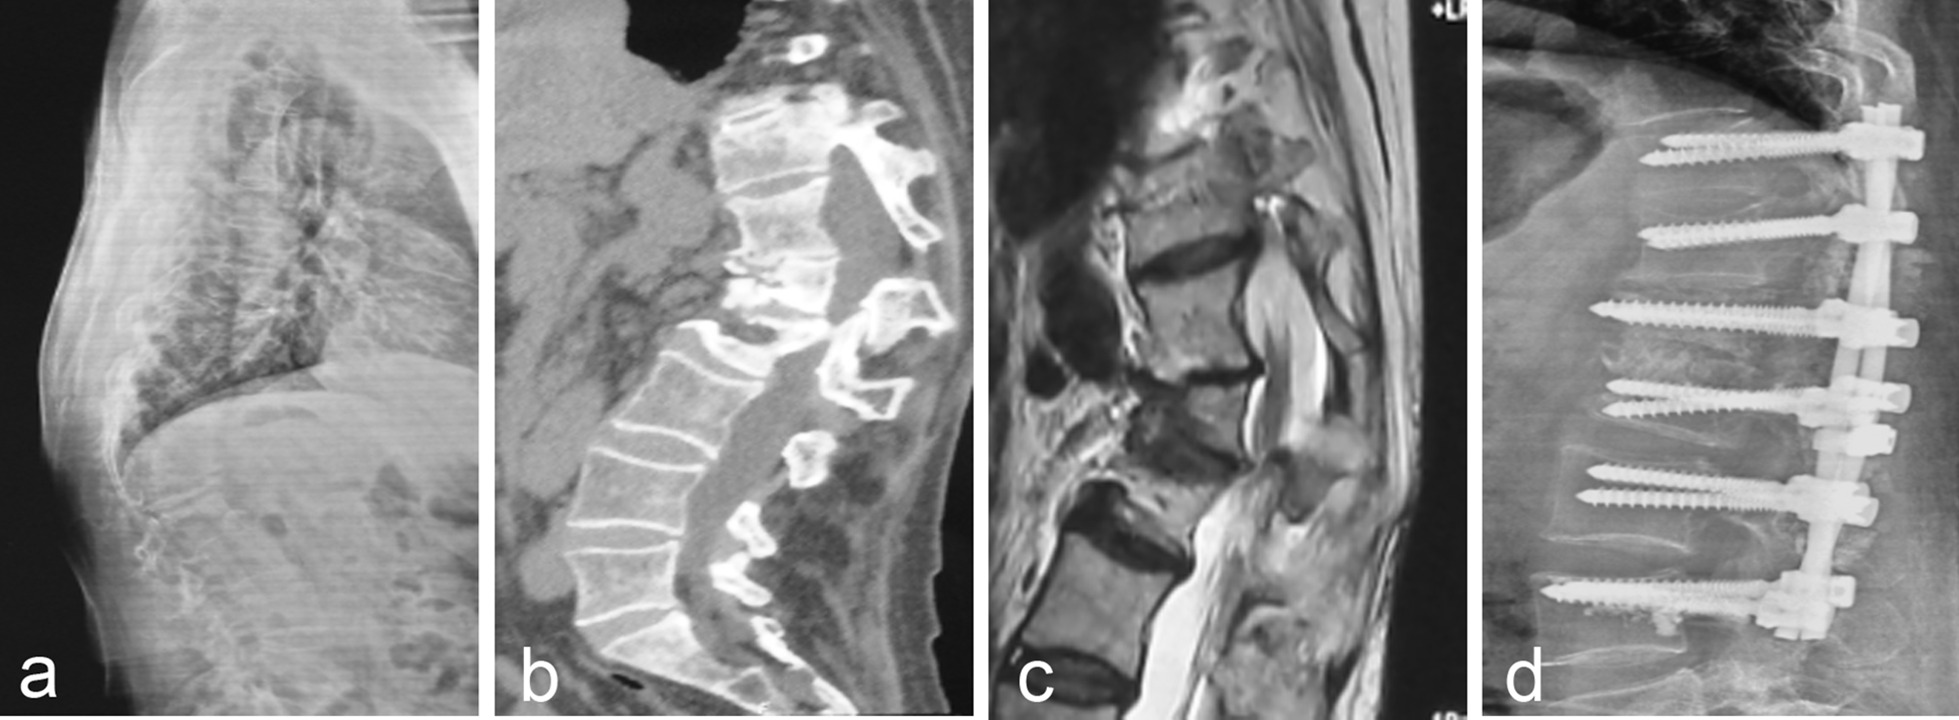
A 55-year-old female patient (Grade V) presented with back pain due to a fall 6 months ago and increased back pain accompanied by numbness and weakness in both lower limbs 2 months ago. a Thoracic X-ray showed wedge-shaped changes of L1 and L2 vertebra with kyphosis of thoracolumbar segment; b CT showed wedge-shaped changes in L1 and L2 vertebrae, kyphosis of the spine, and lumbar spinal stenosis; c Sagittal MRI T2 showed low signal of L1 and L2 vertebrae, kyphosis of thoracolumbar segment, spinal canal stenosis and obvious compression of dural sac; d Postoperative X-ray showed that the patient underwent posterior osteotomy and decompression and reduction combined with internal fixation
